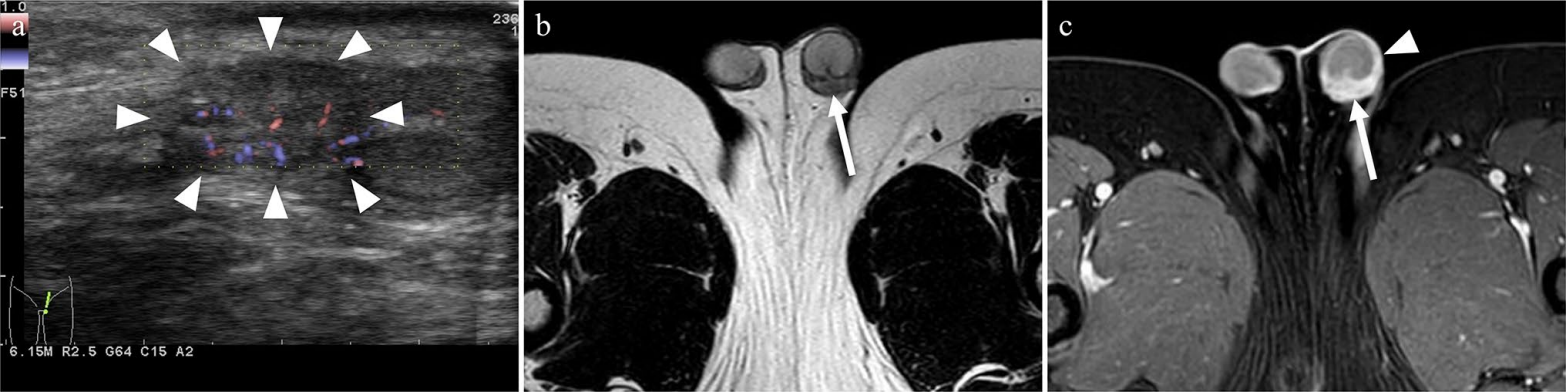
An 8-year-old boy with acute scrotal pain of unknown cause. a Color Doppler US showing an enlarged epididymis (arrowheads) with increased blood flow, corresponding to hyperemia. b On the T2-weighted image, the left epididymis is enlarged (arrow) compared to the opposite side. c On the fat-saturated contrast-enhanced T1-weighted image, the left epididymis appears more intensely enhanced (arrow) than the opposite epididymis, with noticeable enhancement of the scrotal wall (arrowhead). These findings were indicative of epididymitis
